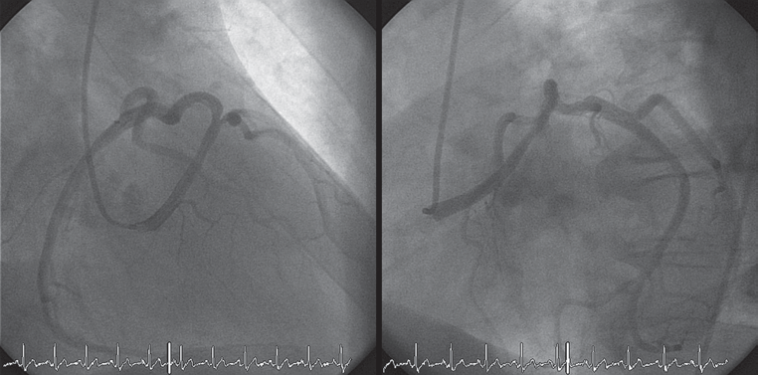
Coronary computed tomography scan of a patient showing an aberrant origin of the *right* coronary artery with a malignant inter-arterial course
